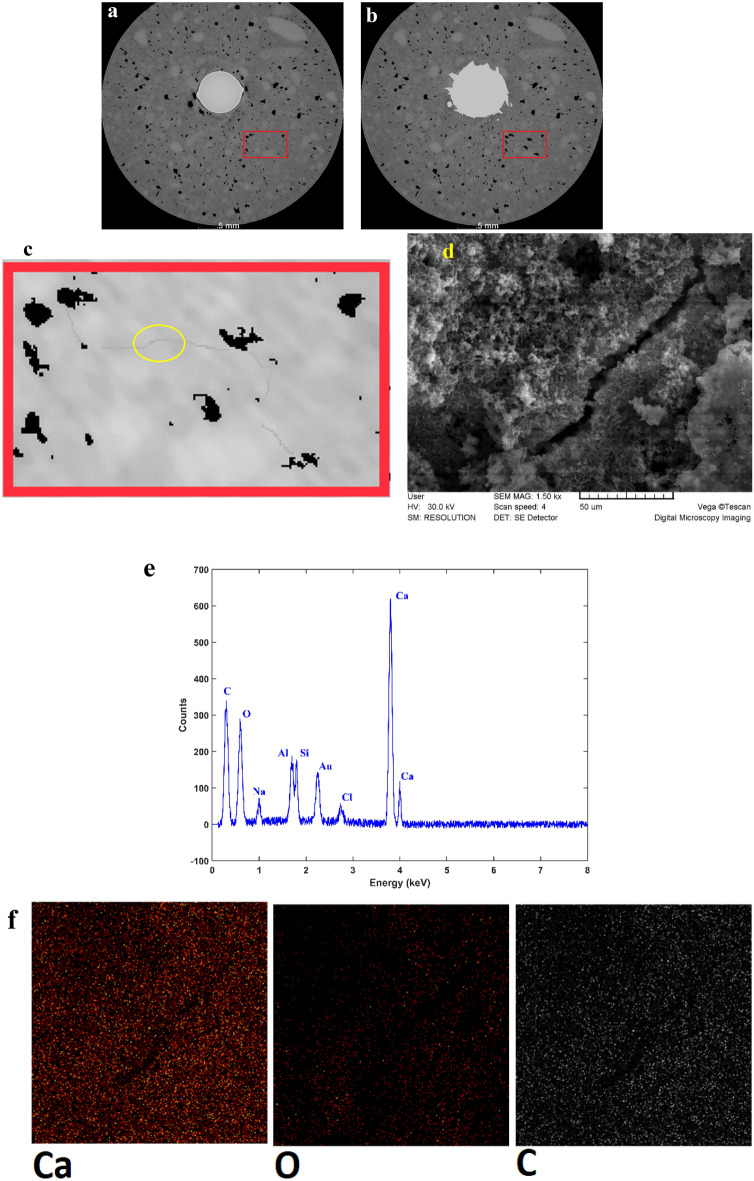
(a) CT scan image of the prototype. (b) CT scan image of the sample after 8 days of accelerated corrosion test. (c) Zipper microcrack development in CT scan images. (d) SEM image of the micro-crack formed. (e) EDS analysis of the specified section. (f) Elemental mapping of the selected section.
